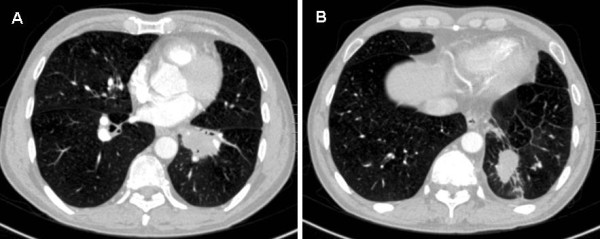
Enhanced CT scan revealing two lobulated masses measuring 30 to 40 mm in diameter in the left inferior lobar bronchus (A) and peripheral tissue of the lower lobe of the left lung (B), respectively.
